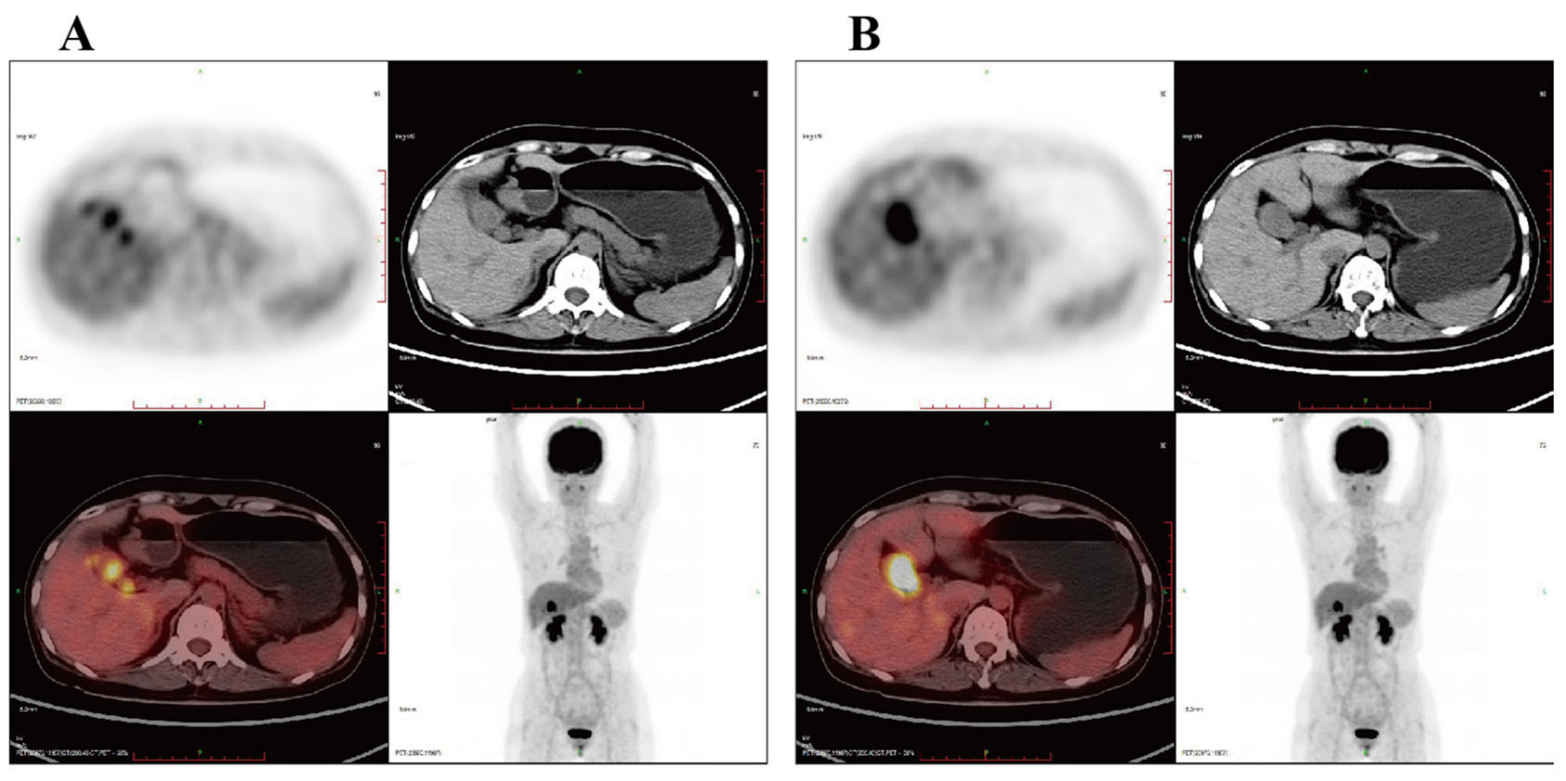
PET/CT suggested that the wall of the gallbladder was thickened to form a soft tissue mass with increased metabolism, which tended to malignant lesions with a high likelihood of gallbladder cancer. (A) The lesion involved the cystic duct and the upper part of the common bile duct; (B) mild dilatation of the intra- and extrahepatic bile ducts. PET/CT, positron emission tomography/computed tomography.
